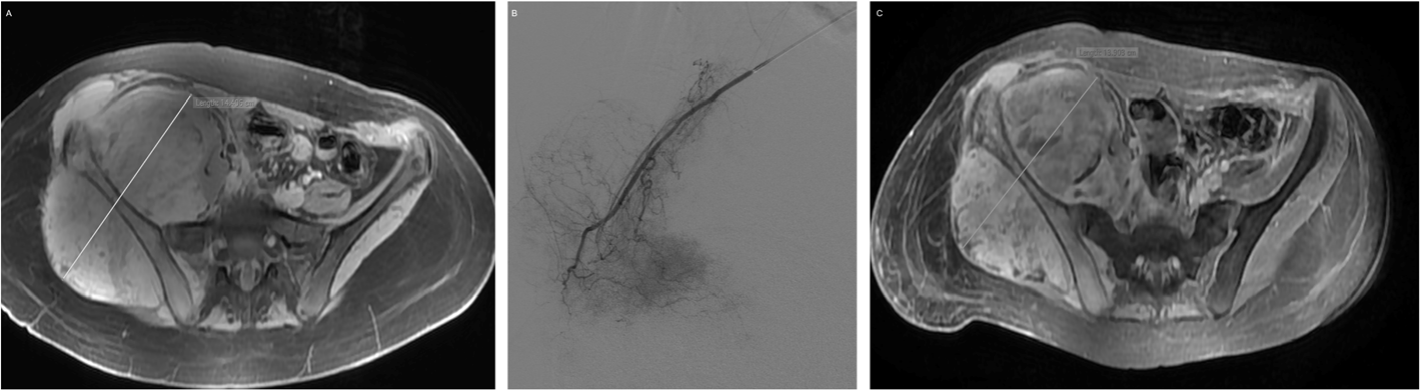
a T1-weighted fat saturated gadolinium (gd) axial MR image demonstrating a large pelvic osteosarcoma (biopsy proven) in a 14-year-old female. b Digital subtraction angiography demonstrating arterial tumor blush from branches of the internal iliac artery. These feeders were embolized with 100–300 DC beads loaded with 100 mg of doxorubicin (DEBDOX-TACE). c T1-weighted fat saturated gadolinium (gd) axial MR image at 1-month demonstrating reduction in tumor size with intra-tumoral necrosis
